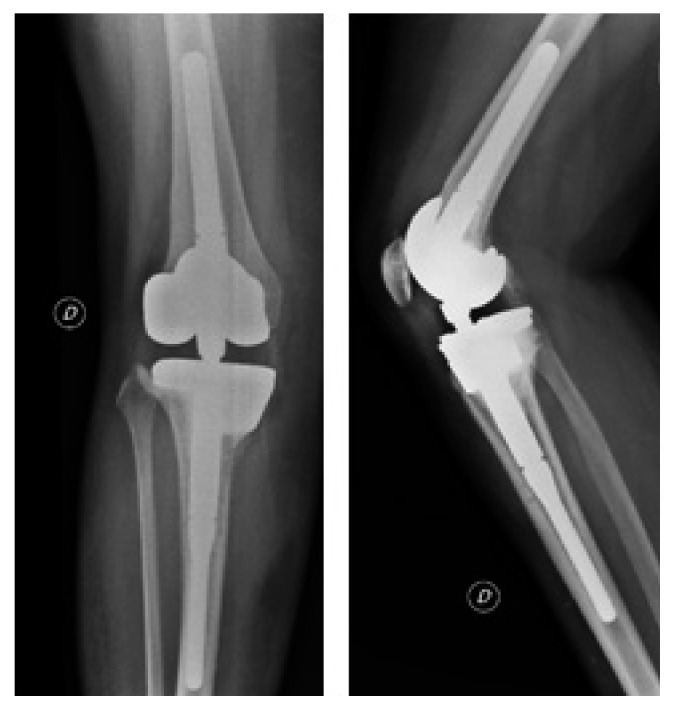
X-rays showing 3 months postoperatively rotating hinge total knee replacement with metal augment.
